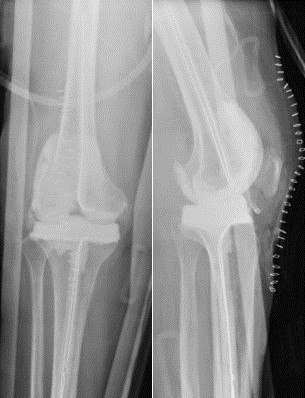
Case 2 anterior–posterior and lateral knee radiographs demonstrating post-surgical changes from the right total knee joint resection and antibiotic impregnated cement and intramedullary rods in the distal femur and proximal tibia.
